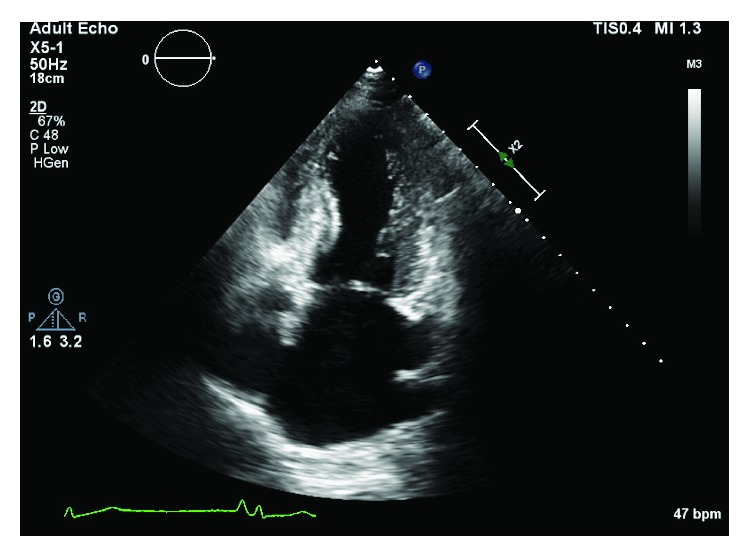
Echocardiogram (2-chamber long-axis view) showing the apical ballooning suggestive of takotsubo cardiomyopathy.
